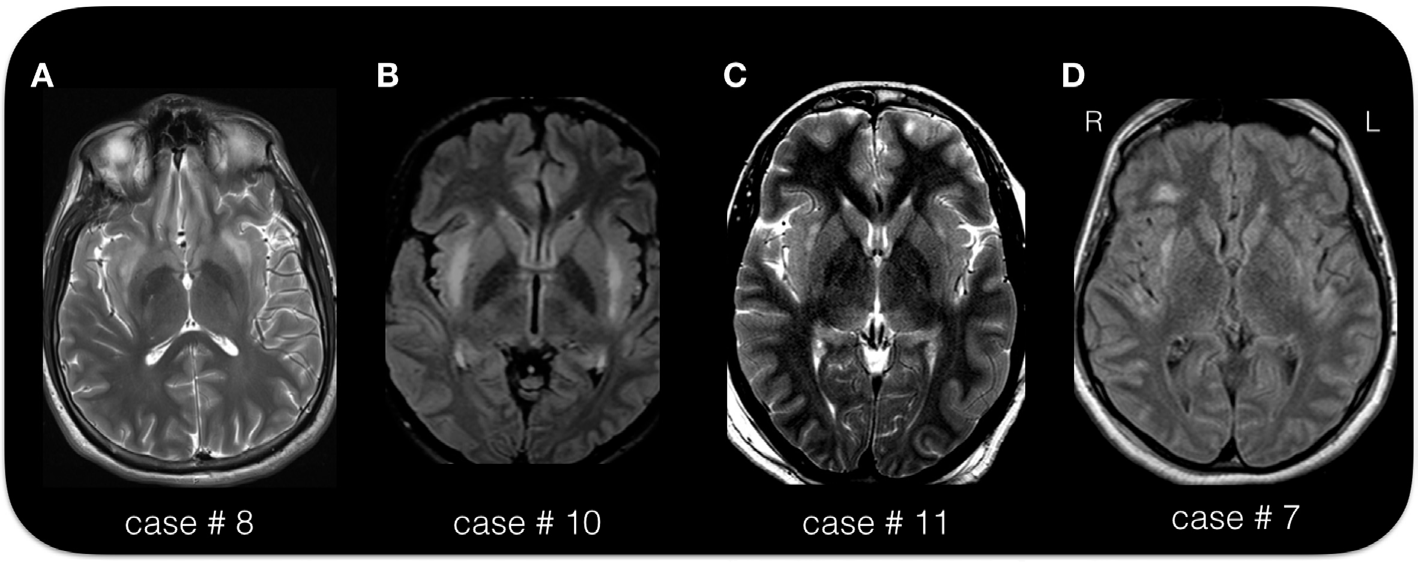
The “claustrum sign” in four patients. (A) Axial T2 sequence in case # 8, 25-year-old male, acquired 15 days after status epilepticus (SE) onset. (B) Axial FLAIR sequence in case # 10, 19-year-old female, acquired 6 days after SE onset. (C) Axial T2 image in case # 11, 33-year-old female, acquired 21 days after SE onset. (D) Axial FLAIR image in case # 7, 38-year-old female, acquired 25 days after SE onset. Note the hyperintense appearance of the capsula esterna/claustrum region in the four cases. Despite the different MRI scanner and image parameters, the hyperintensity of the claustrum is easily recognizable in each case. The exact location and extension of the signal alteration varies across the four cases. In all patients is present a bilateral involvement. Note the signal alteration extending to the insular/peri-insular cortex and subcortical white matter in panel (D).
